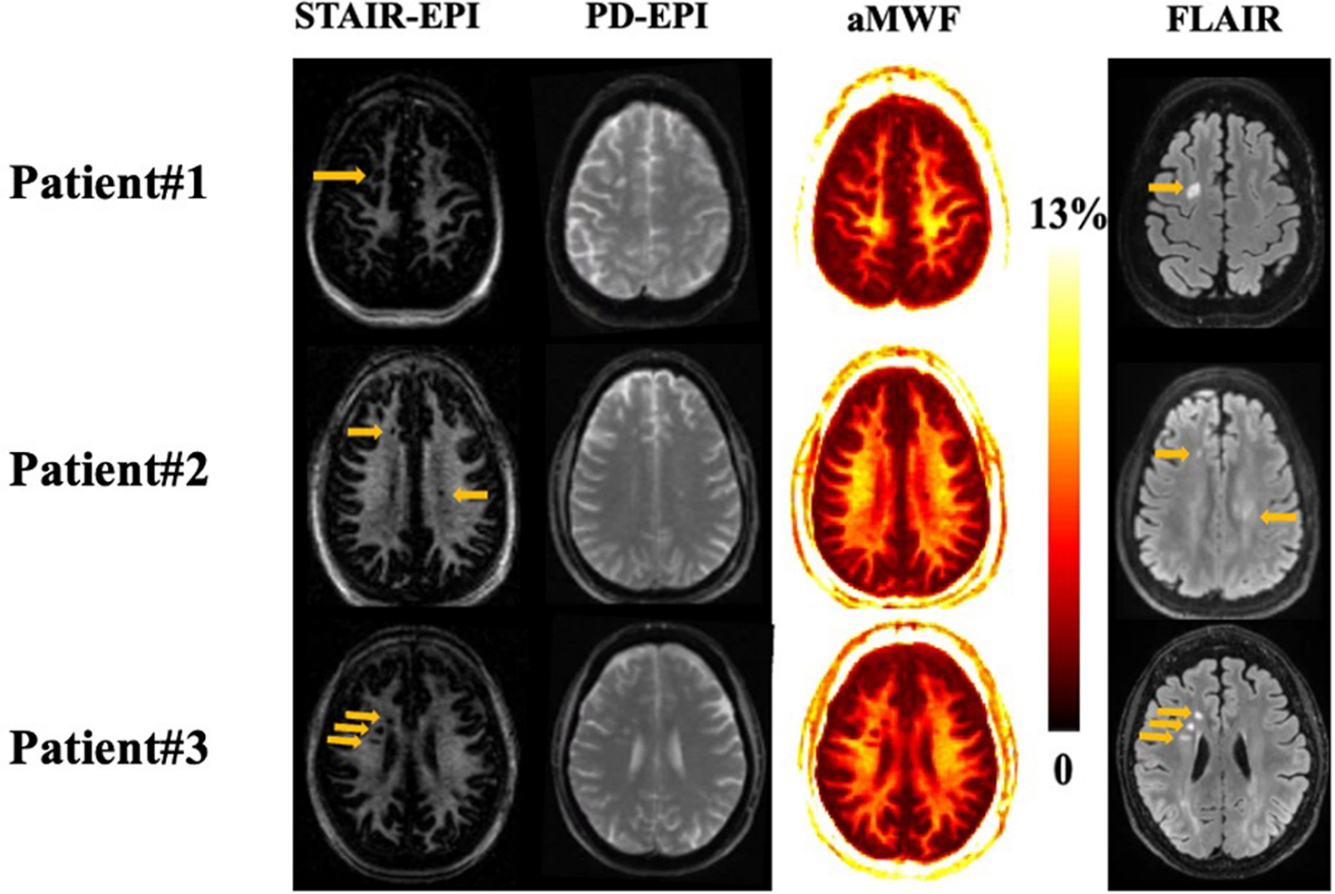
Representative STAIR-EPI (first column), PD-EPI (second column), and aMWF (third column), as well as *T*_2_-FLAIR (fourth column) from three patients with MS (patient #1 is a 57-year-old female, patient #2 is a 52-year-old male, and patient #3 is a 67-year-old female). Hyperintense lesions detected on *T*_2_-FLAIR images (arrows) show a signal loss on the corresponding myelin water images and aMWF maps.
